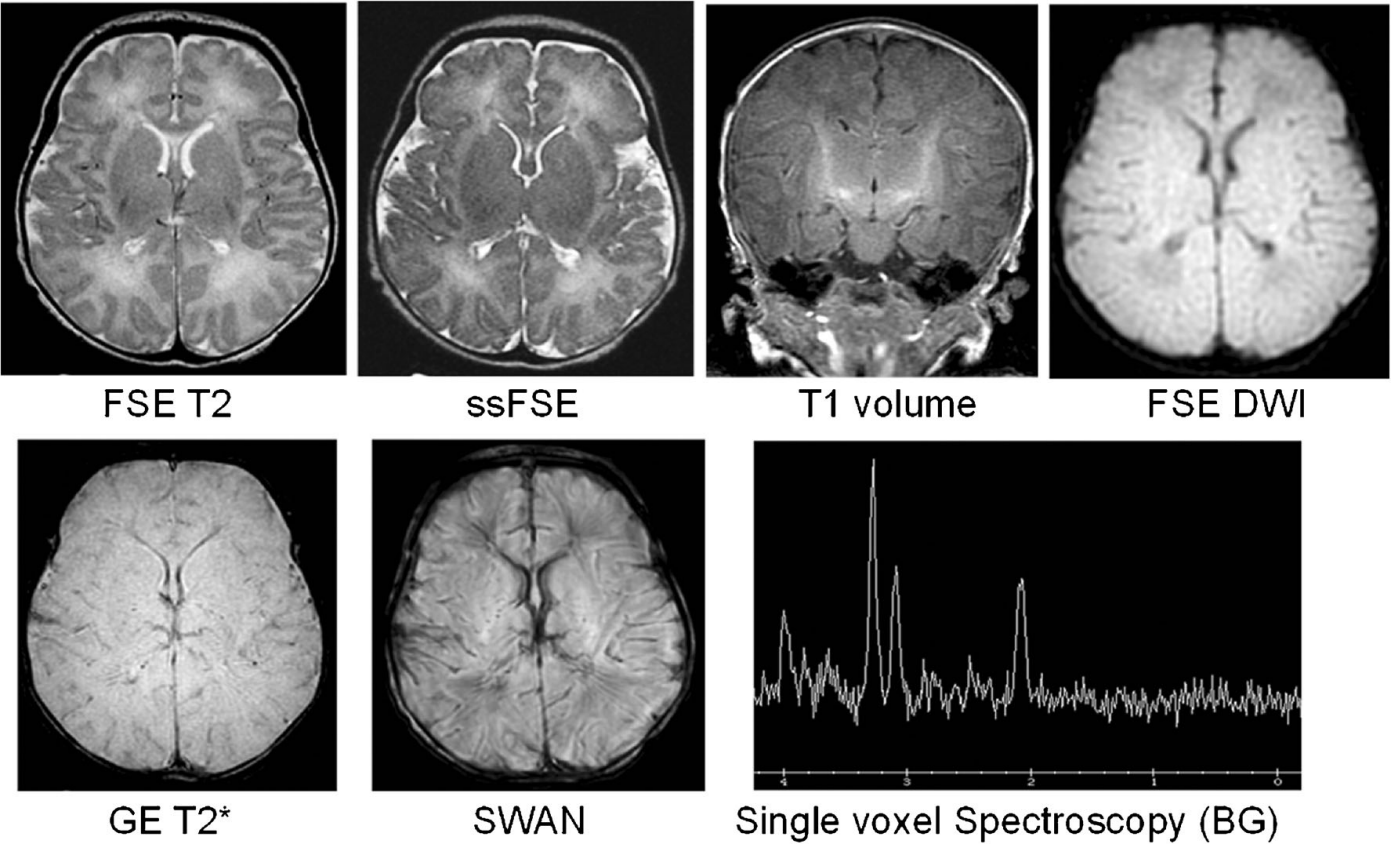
Representative MR images from a normal baby born at term showing the range of routine sequences acquired in cases 20-49 in this study
